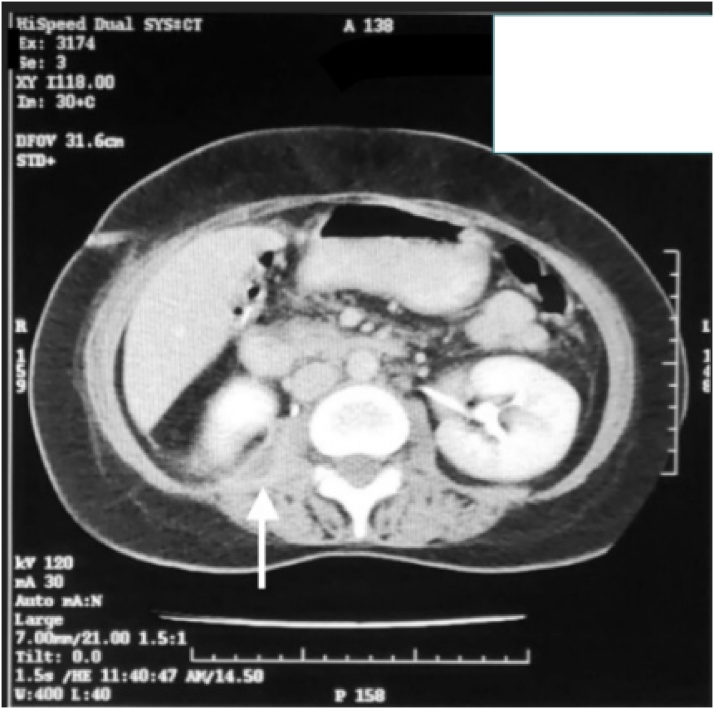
CT showing abdominal collection in the right psoas muscle.
